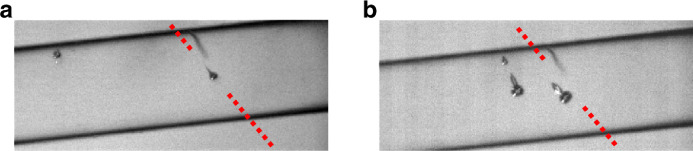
Top view images of initial microbubble cavitation response, at *t* ≈ 11.4 μs, to a focused ultrasound burst of peak negative pressure = 1.5 MPa, with the propagation axis depicted by the red dashed line, for (a) a relatively isolated microbubble located centrally within the capillary, and (b) three microbubbles, two located centrally within the capillary and one closer to the wall. Flow within the capillary is left to right. Scale is provided by the internal diameter of 500 μm, and a flaw on the capillary (*white arrowhead*) acts as a convenient fiducial.
